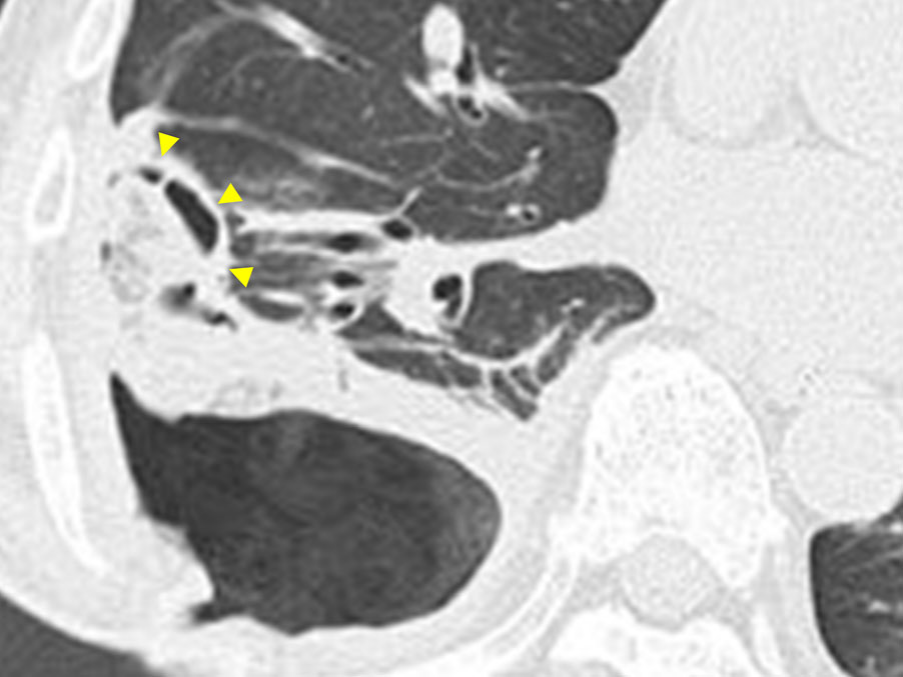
Computed tomography of the chest at 1 month after the thoracostomy. The subpleural lung parenchyma was necrosed along the subpleural line (arrowheads).
